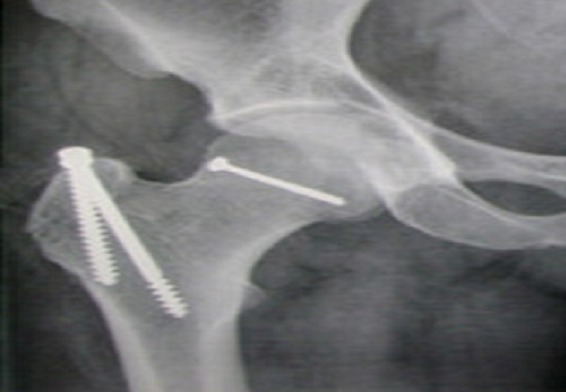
Radiographie de la hanche de face à 18 mois de recul sans signes d'ostéonécrose ni d'arthrose, la trochantérotomie étant consolidée
